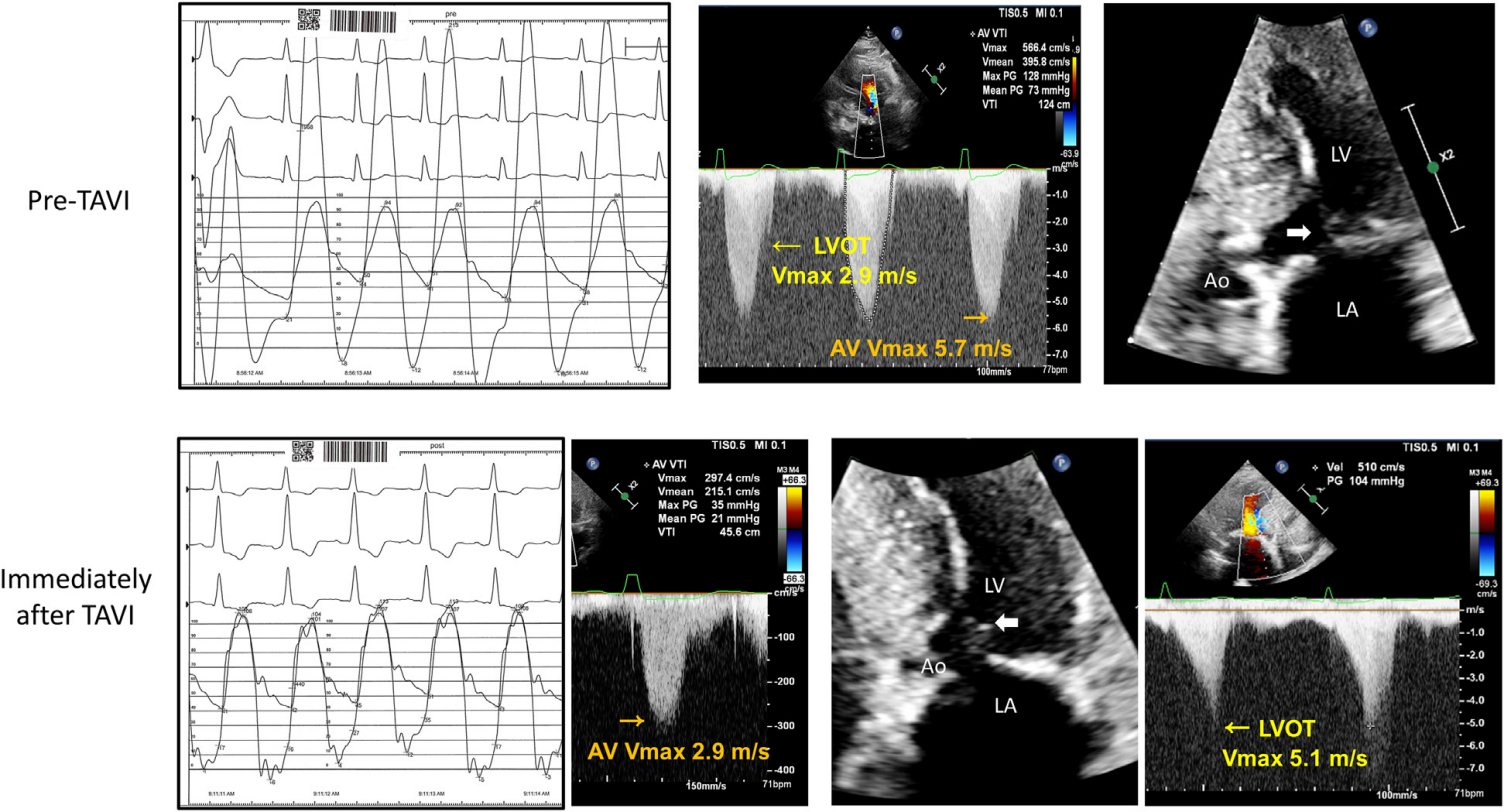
Invasive and Echocardiographic Hemodynamic Assessment Before and After TAVI Before transcatheter aortic valve implantation (TAVI), the systolic anterior motion of the mitral leaflet (SAM, arrow) was not prominent and left ventricular outflow tract (LVOT) flow velocity was not significantly high. Immediately after TAVI, no pressure gradient was present between the left ventricle (LV) and aorta (Ao), but follow-up echocardiography done several days after TAVI showed septal contact of SAM (arrow) resulting in a markedly increased pressure gradient across the LVOT. LA = atrium; Vmax = maximal velocity.
